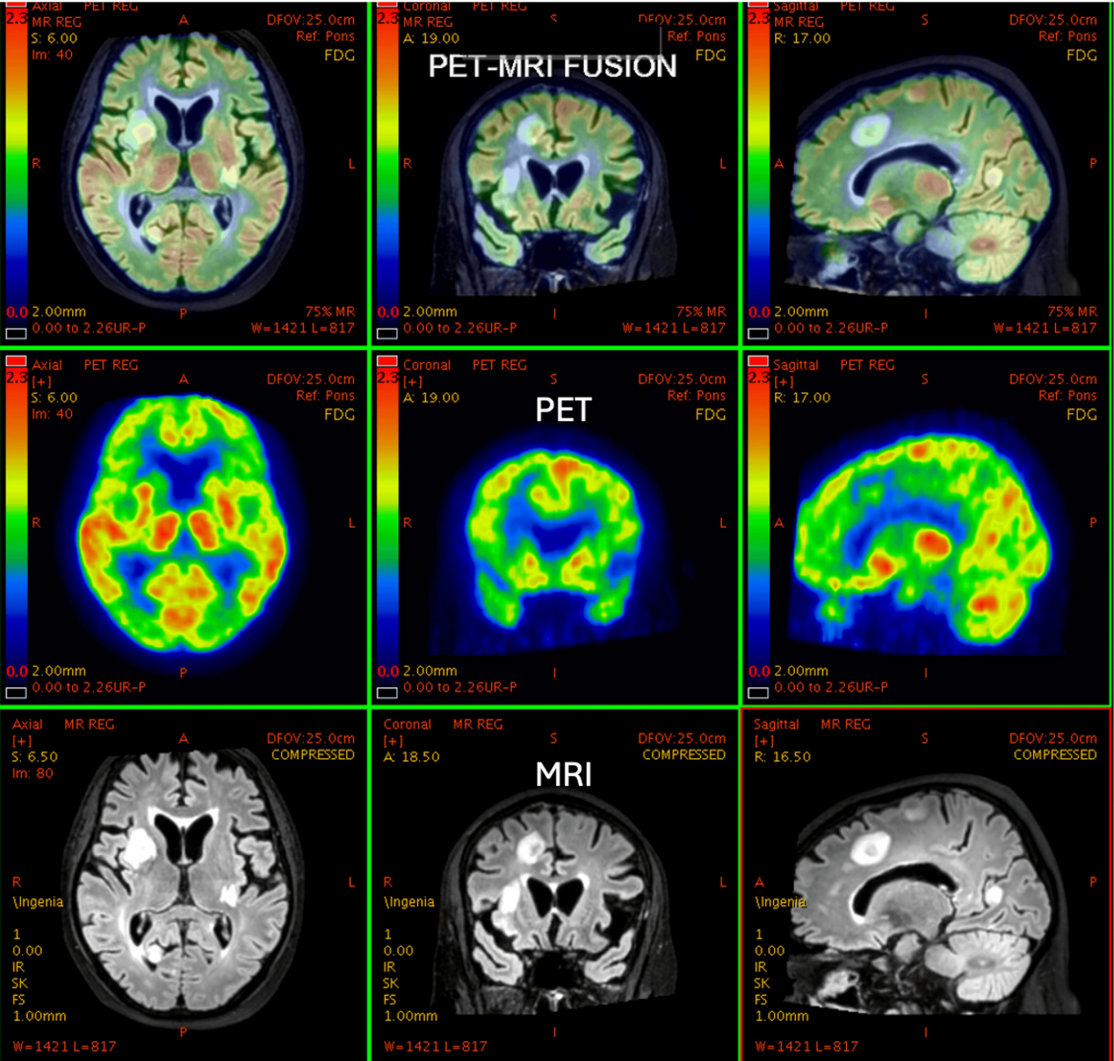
51-year-old male, K/c/o Ca prostate with brain metastasis. Post-radiotherapy to the brain. PET-MRI shows a reduction in metabolic activity of metastatic brain lesions, suggesting a response to therapy. PET: positron emission tomography; MRI: magnetic resonance imaging
